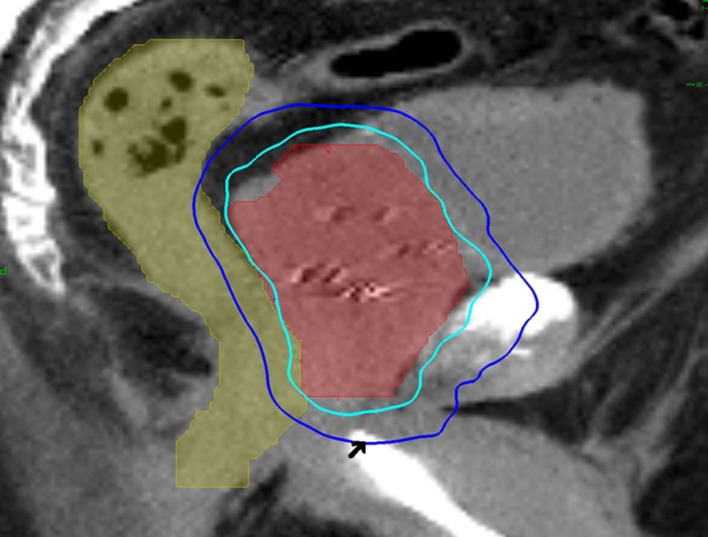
A 60-year-old man with intermediate risk prostate cancer had cardiac disease and an internal defibrillator, which precluded magnetic resonance imaging for treatment planning. Thus, he was treated with urethrogram-directed SBRT: treatment planning sagittal computed tomography urethrogram images demonstrating the prostate (red) and rectum (green) are shown. Arrowhead marks the “beak” of the urethrogram. Isodose lines shown as follows: 100% of the prescription dose (light blue line) and 50% of the prescription dose (dark blue line).
